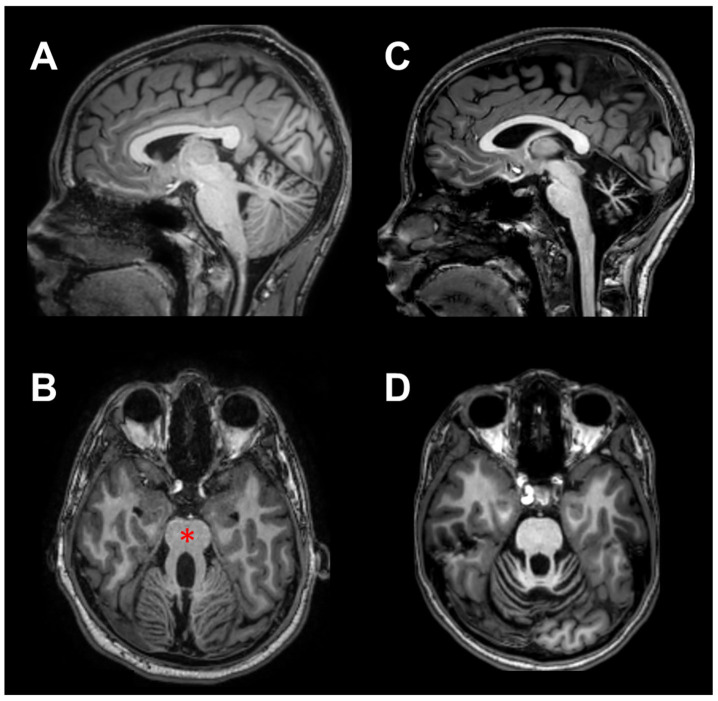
MRI scans of two representative ataxic children, in sagittal (A,C) and axial (B,D) views. In (A,B), scans of a child with non-progressive ataxia (NonP) show the typical vermian hypoplasia (“molar tooth” sign, red asterisk). In (C,D), scans of a child suffering from slow-progressive ataxia (SlowP) with evident generalized cerebellar atrophy are presented.
